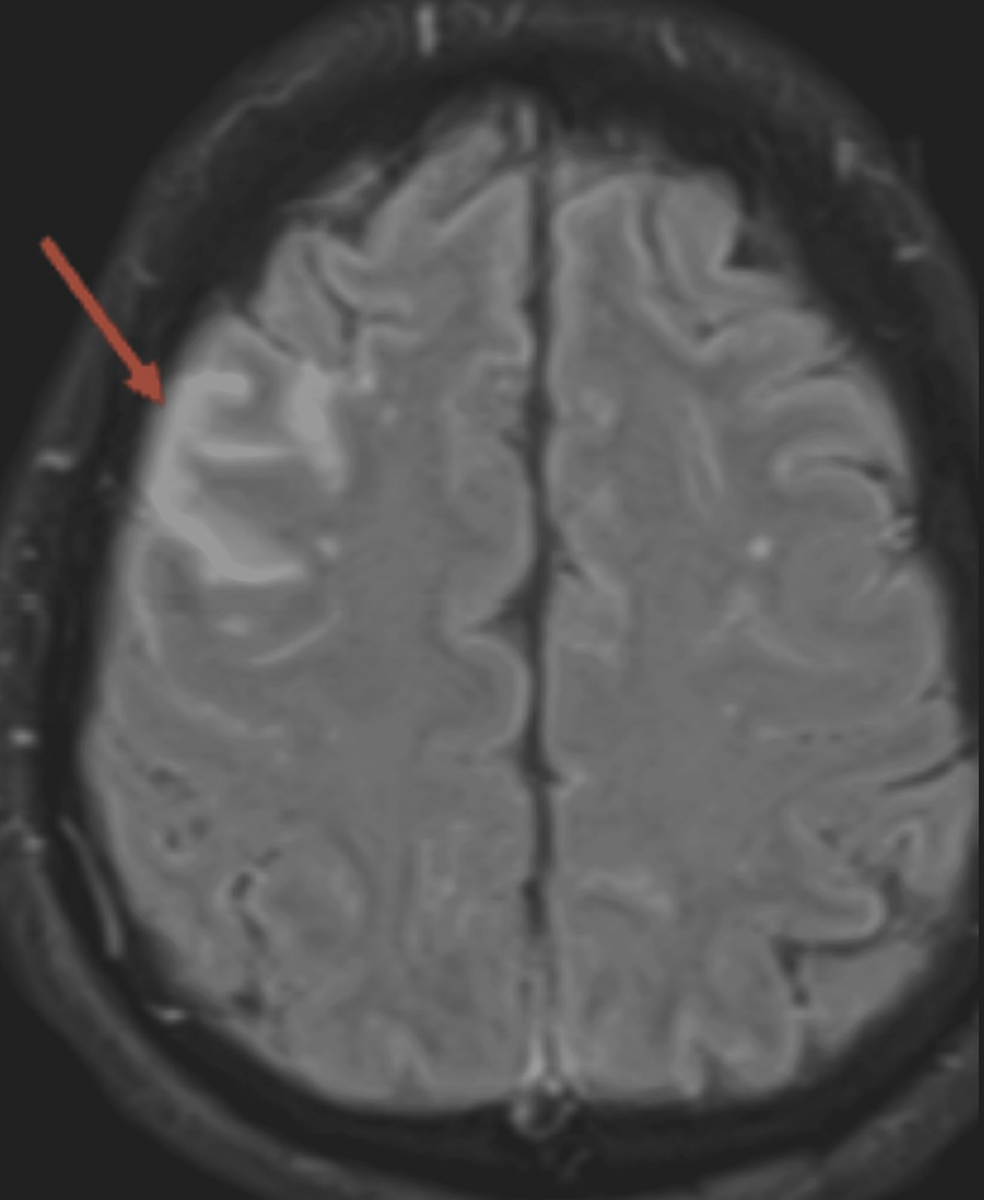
Brain MRI showing curvilinear FLAIR signal abnormality in gyriform pattern in the right frontal lobe in a patient with GAD and VGKC antibodies (red arrow). FLAIR: fluid-attenuated inversion recovery; GAD: glutamic acid decarboxylase; VGKC: voltage-gated potassium channel.
